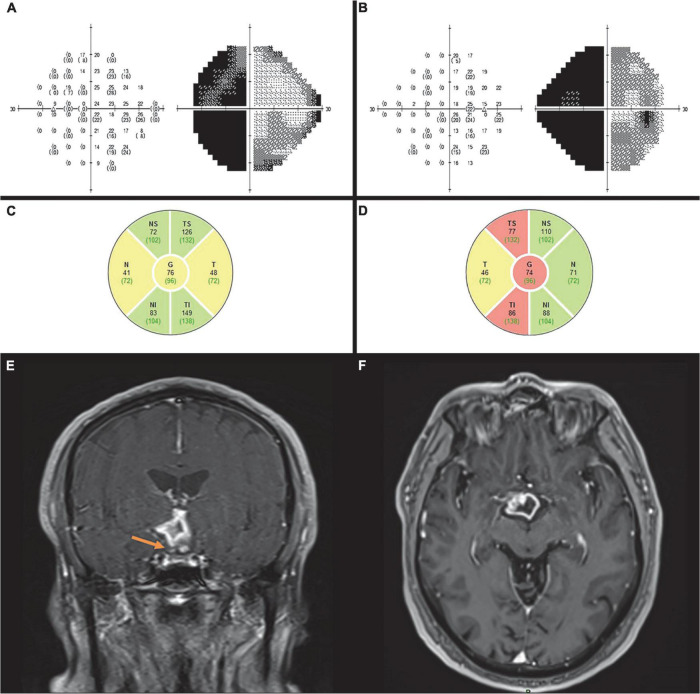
Case 2. Automated perimetry shows left homonymous hemianopia in the left (A) and right (B) eye. OCT demonstrates RNFL thinning in the left nasal and temporal (C) and right superior and inferior quadrants (D). MRI T1 coronal (E) and axial imaging. (F) After contrast show a ring enhancing lesion involving the hypothalamus and right optic tract (arrow) causing posterior compression of the chiasm consistent with low -grade glioma.
